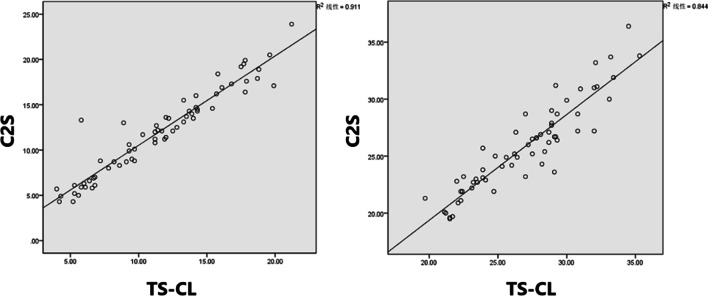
C2S was strongly linearly correlated with TS-CL on standard lateral radiographs (*R*^2^ = 0.911, non-kyphosis) (*R*^2^ = 0.844, kyphosis)
